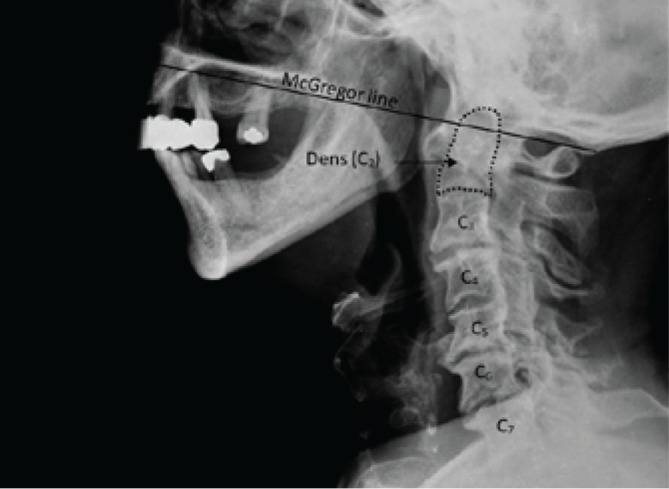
Conventional radiography of the neck - Lateral view (and after the interpretation of the image - Extensive degenerative changes involving the C3, C4 and C5 vertebral bodies, as well as fusion of the apophyseal joints C2–C3 and C4–C5.
